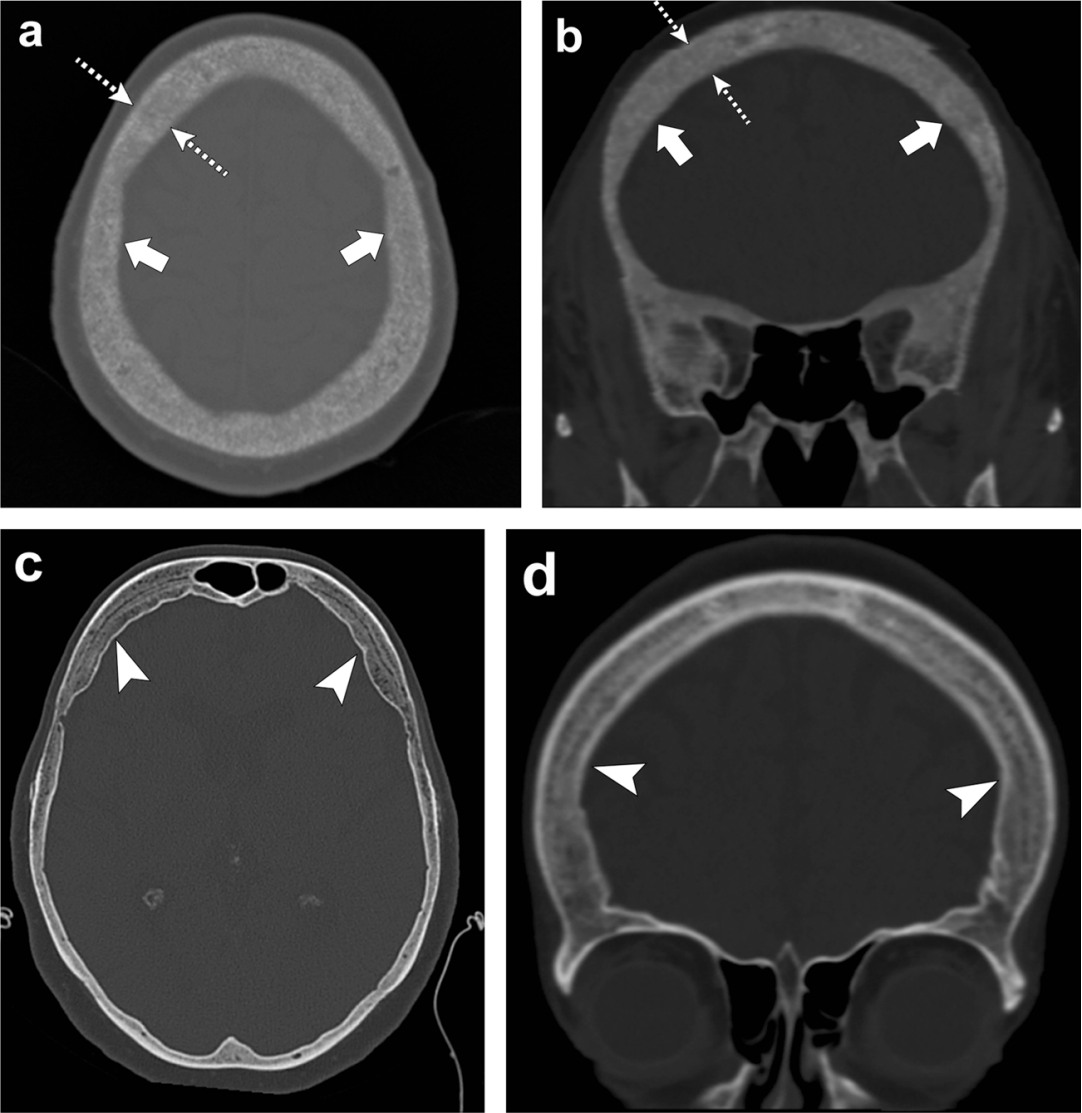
Renal osteodystrophy and osteopenia. *Patient 1:* Axial (a) and coronal (b) head CT images depict granular de-ossification with a “pepper pot” appearance (*thick arrows*) and loss of distinction of the inner and outer tables (*dashed arrows*) in this patient with renal osteodystrophy. *Patient 2:* Axial (c) and coronal (d) head CT images show demineralisation of the skull (*arrowheads*) in this patient with osteopenia. Note the relative preservation of the distinction of the inner and outer tables in osteopenia
